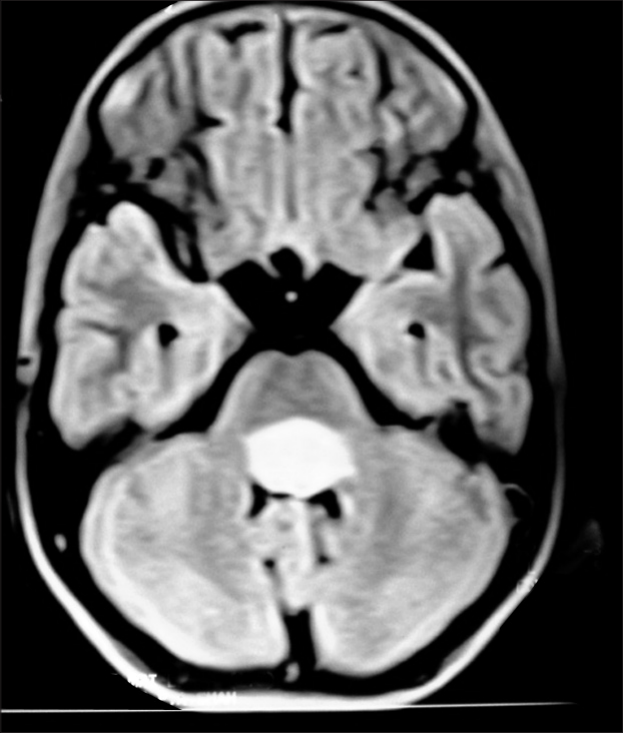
Fourth ventricular ependymoma on T1W axial MRI brain of a 10 year old brother, whose 13 year old sister Figure 7 has right diencphalic oligodendroglioma
